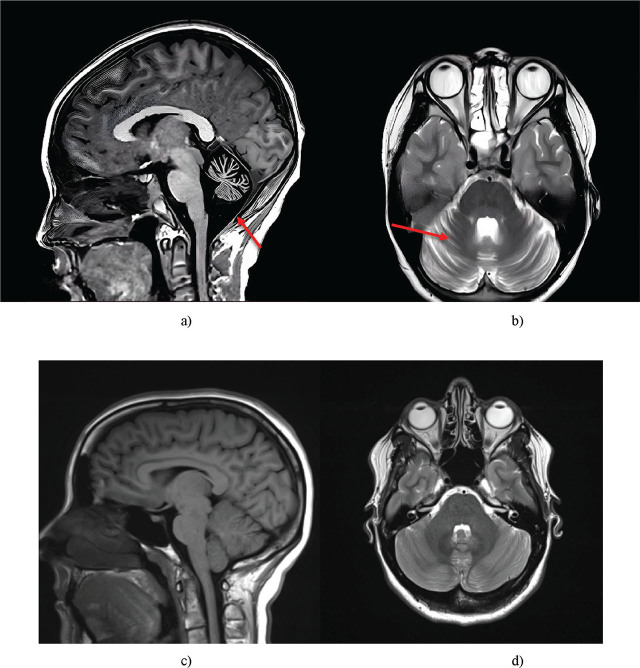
Brain MRI of the middle child (Patient 2): a) sagittal; b) axial sections*. Healthy brain: c) sagittal; d) axial slices. The pathological features observed include moderate vermian thinning and cerebellar hemispheric subatrophy with moderate sulcal widening. *Source:* a-b were obtained during the study and c-d are reproduced from [34].
